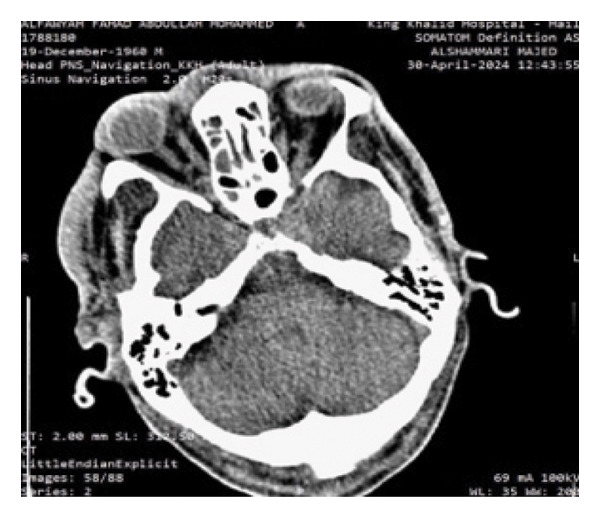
Axial CT scan showing right‐sided sinusitis with bony erosion of the anterior and superior orbital walls.
